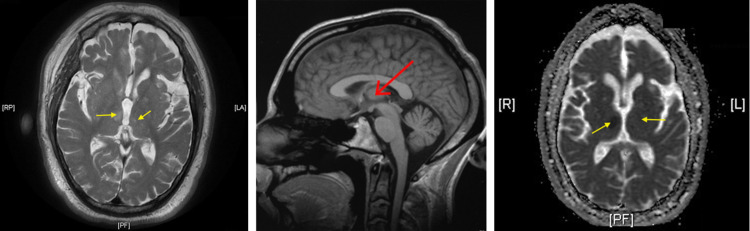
MRI demonstrating right greater than left bilateral thalamic infarcts (yellow arrows). The left panel is the axial T2 FLAIR image, the center panel shows the infarct on sagittal view (red arrow), and the right panel is the ADC image. MRI, magnetic resonance imaging; FLAIR, fluid-attenuated image recovery; ADC, apparent diffusion coefficient
